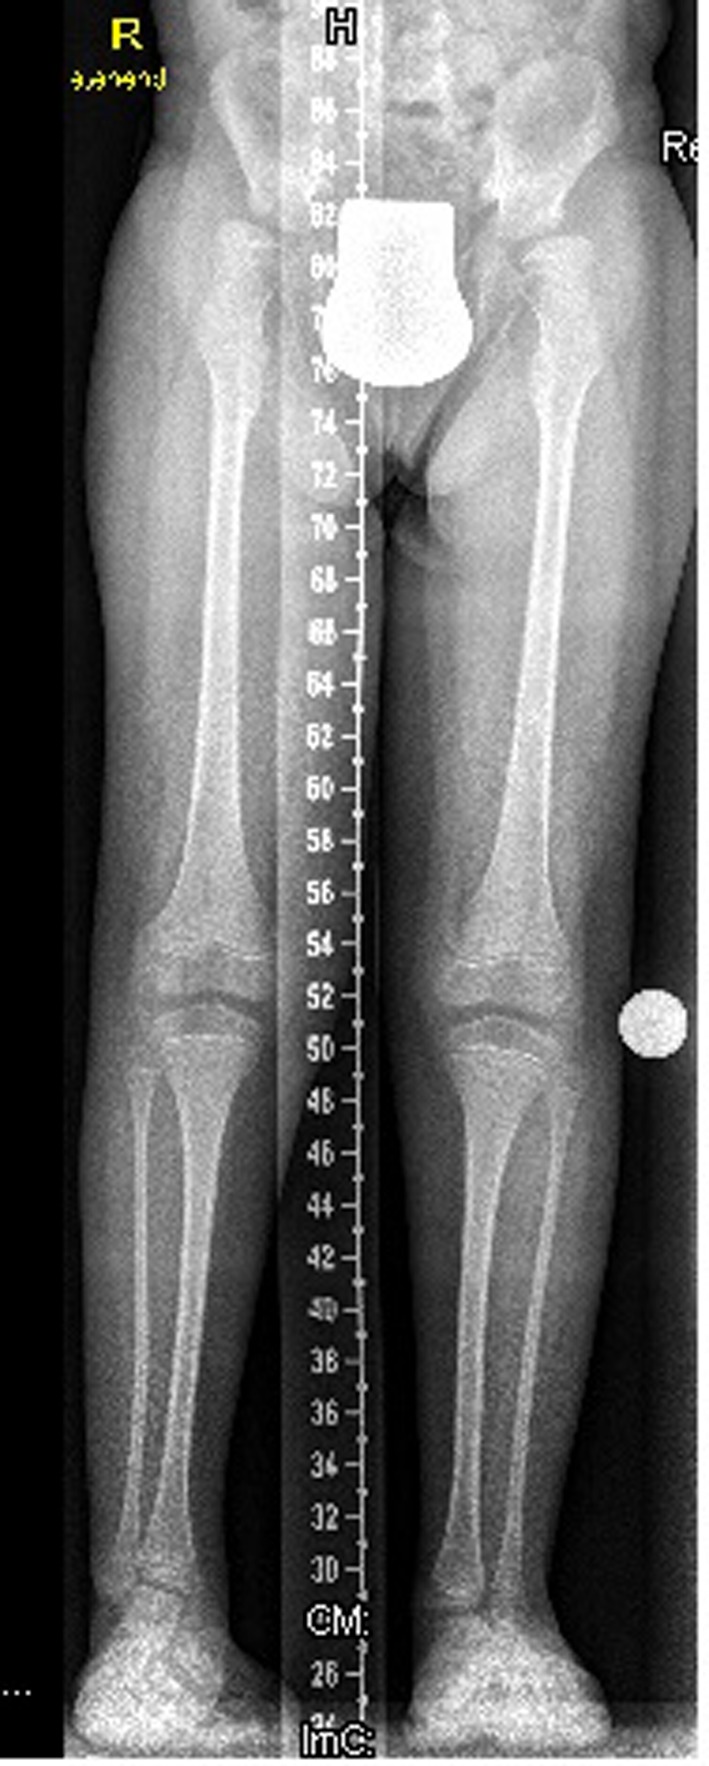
Lower limb standing radiograph showed a hypoplastic iliac bones, severe coxa valga, associated moderate subluxation of the hip because of incomplete development of the acetabulae with over-tubulation of the long bones.
